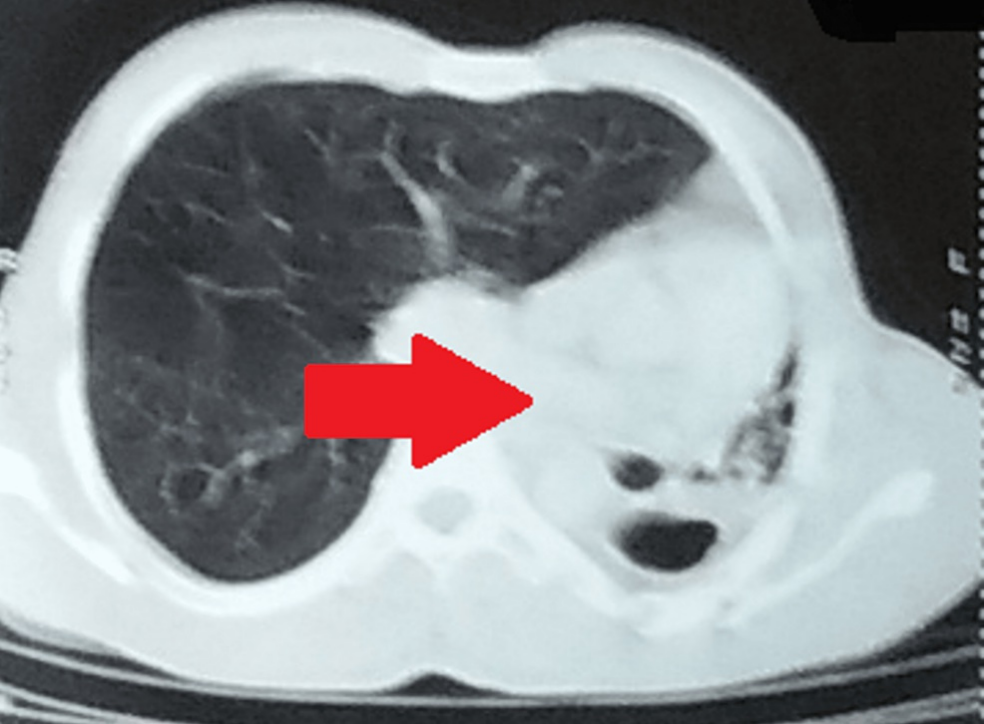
CT chest showing the collapse of the left lung
